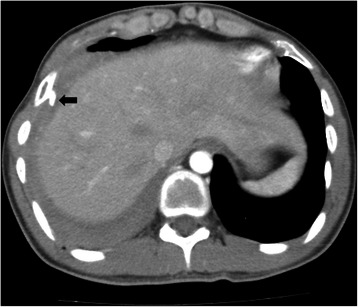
Chest computed tomography scan showing pleural effusion In the right hemithorax. The black arrow indicates a protruding rib spiculation
